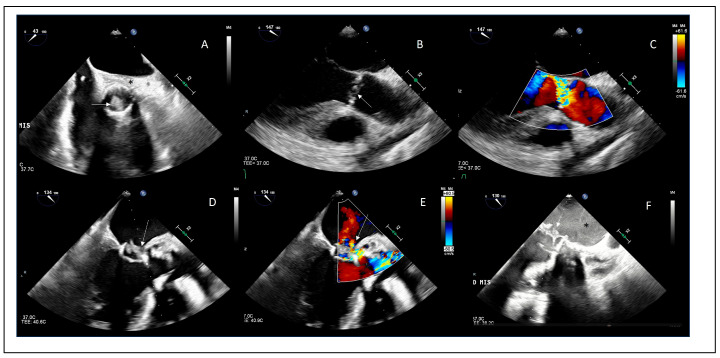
Transesophageal echocardiography findings of intracardiac complications of infective endocarditis. Panel (A). Aortic abscess. Two-dimensional transesophageal echocardiography showing a periprosthetic aortic abscess (asterisk) in a patient with a biological aortic valve prosthesis. The arrow indicates a rounded, sessile vegetation adherent to the prosthetic leaflets, consistent with prosthetic valve endocarditis. Panel (B). Leaflet perforation. Two-dimensional transesophageal echocardiography demonstrating a perforation of a native aortic valve leaflet secondary to infective endocarditis, indicated by the arrow. Panel (C). Leaflet perforation. Corresponding color Doppler imaging confirms severe aortic regurgitation with an eccentric jet, resulting from IE-related leaflet perforation. Panel (D). Fistula. Shows two-dimensional transoesophageal echocardiography in a long-axis view, demonstrating a perivalvular abscess secondary to infective endocarditis involving the mitro-aortic continuity. The abscess cavity extends into the fibrous intervalvular region and is complicated by the formation of a fistulous communication between the left ventricular outflow tract (LVOT) and the left atrium (arrow). Panel (E). Fistula. Depicts the same view with color Doppler imaging, clearly visualizing turbulent systolic flow across the fistulous tract from the LVOT into the left atrium, confirming the presence of a hemodynamically significant intracardiac fistula (arrow) as a complication of infective endocarditis. Panel (F). Severe prosthetic dysfunction. Two-dimensional transoesophageal echocardiography demonstrates severe dysfunction of the mitral bioprosthesis secondary to a large vegetation adherent to the atrial side of a prosthetic mitral leaflet (arrow). The vegetation results in marked obstruction of transprosthetic flow, leading to severe bioprosthetic mitral stenosis. Prominent spontaneous echo contrast (“smoke effect”) is evident shown by the asterisk within the left atrium (asterisk), reflecting severe blood flow stasis due to critical valve obstruction.
